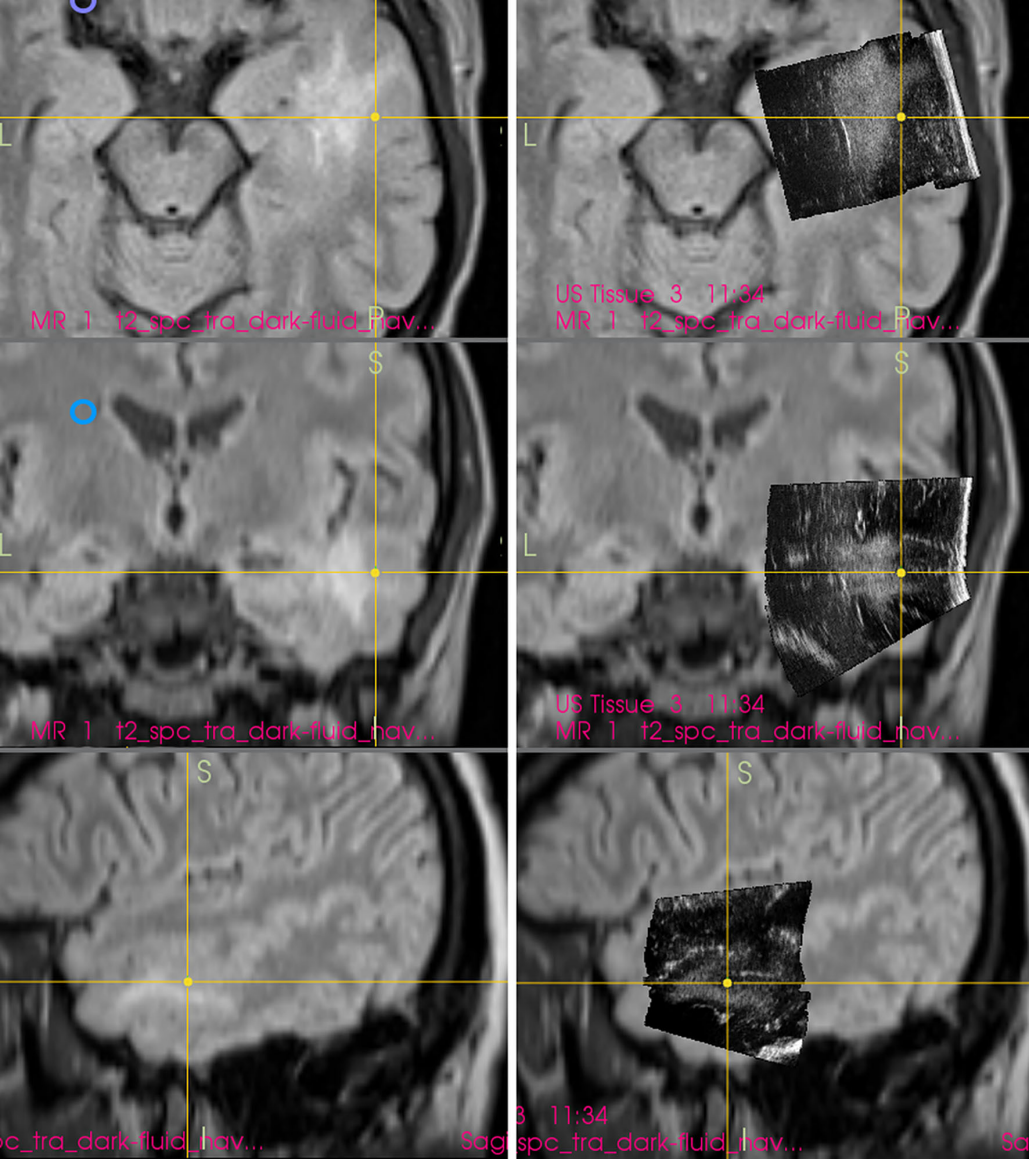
Right-sided temporal grade II astrocytoma (Left column) preoperative navigation 3D FLAIR MRI sequence (1.5-Tesla MRI scanner) (Right column) pre-resectional 3D IOUS image fused with navigation FLAIR MRI sequence. Note that the hyperechoic tumor tissue is better visualized on IOUS image comparing to MRI. 3D, three-dimensional; FLAIR, fluid attenuated inversion recovery; MRI, magnetic resonance imaging; IOUS, intraoperative ultrasound.
